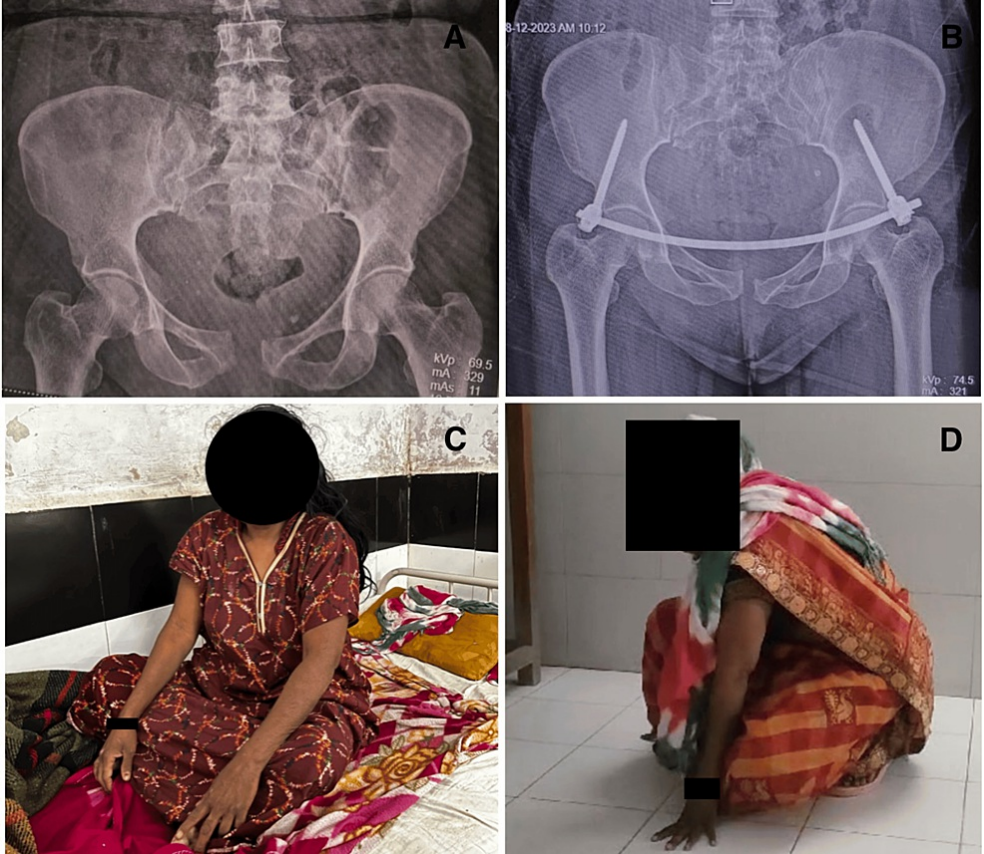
(A) Preoperative X-ray showing APC-II fracture; (B) postoperative X-ray INFIX; the patient sitting cross-legged (C) and squatting (D) six months after surgery APC: anteroposterior compression; INFIX: internal pelvic fixator
